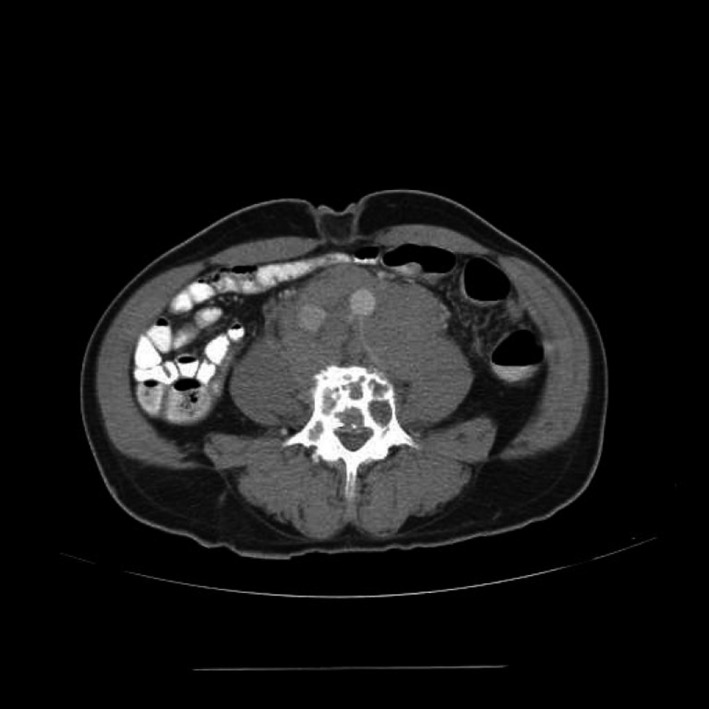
Contrast‐enhanced axial CT of the abdomen showing extensive retroperitoneal adenopathy encasing the abdominal aorta and inferior vena cava, displacing them anteriorly. Multiple lytic lesions are seen on the lumbar vertebra body
